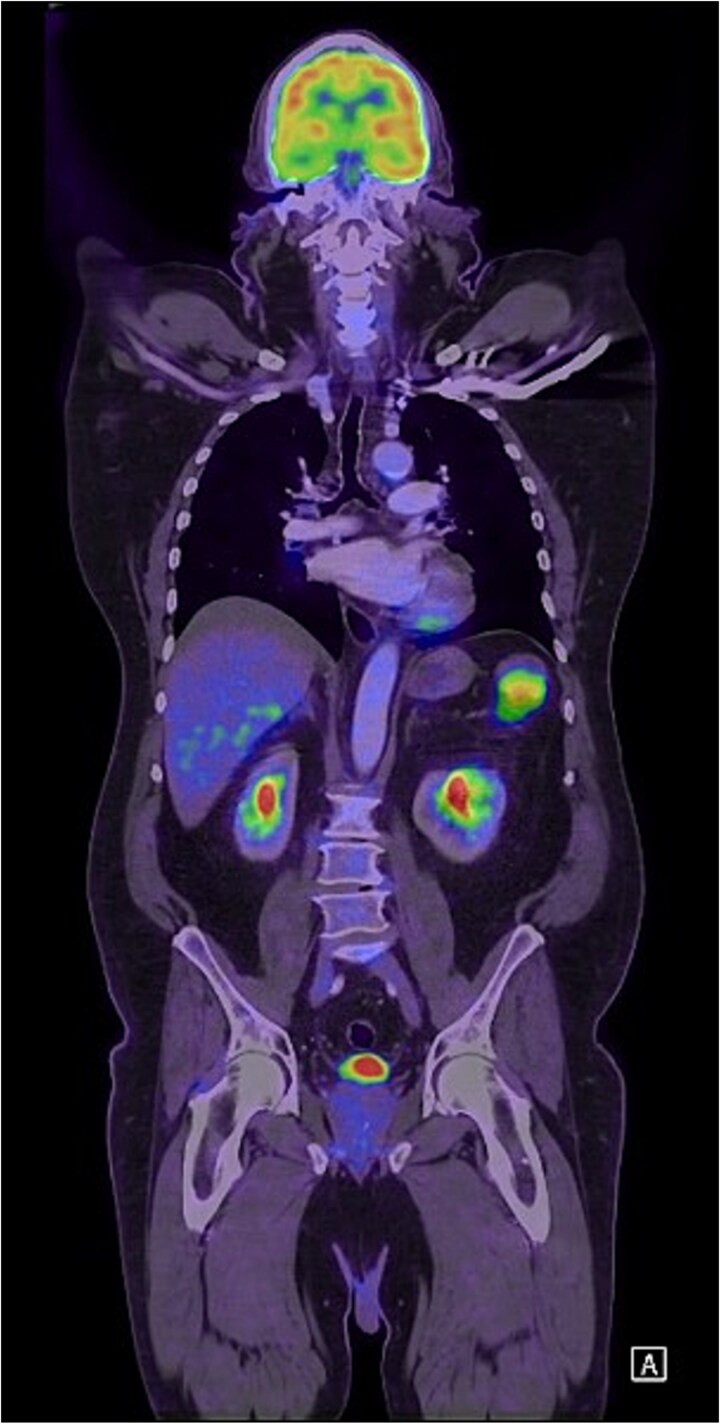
Coronal FDG PET scan of the head, neck, chest, and abdomen demonstrating a PET-avid LUQ lesion.
